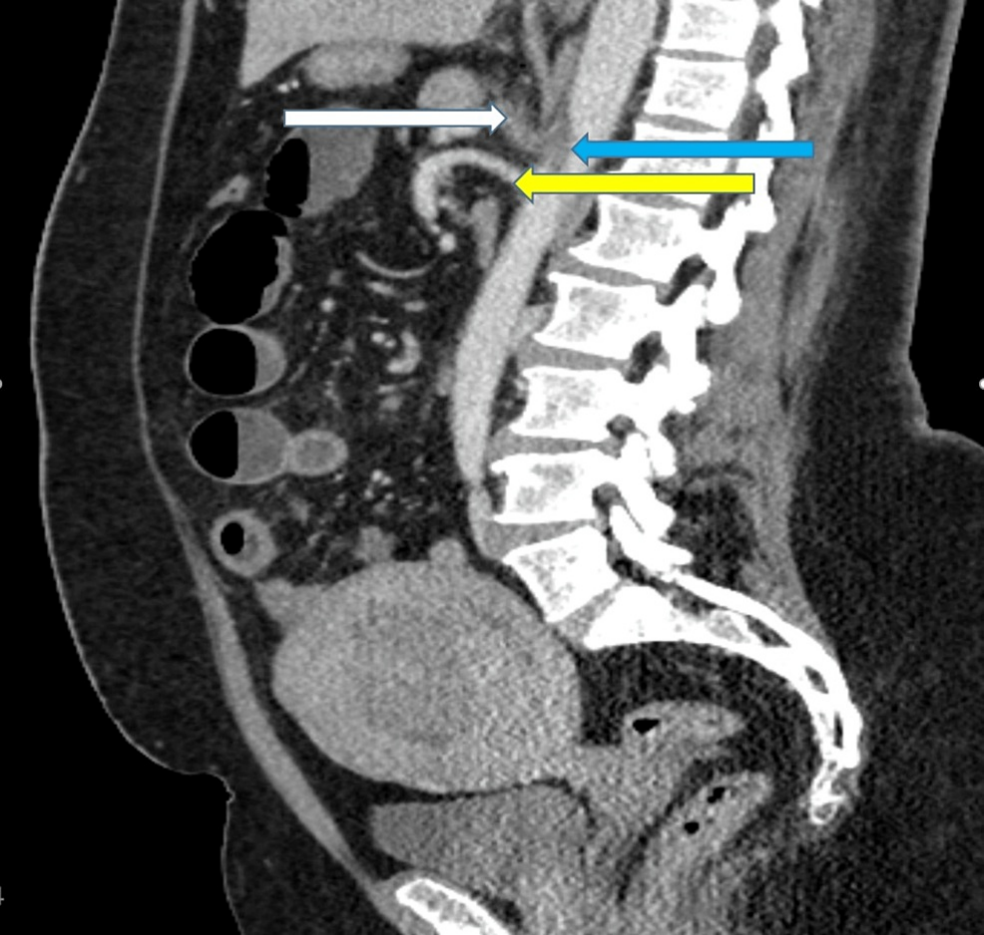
Abdominal CTA image (sagittal view) showing the aortic thrombus (blue arrow) centered on and completely occluding the origin of the celiac trunk artery (white arrow) without involving the superior mesenteric artery (yellow arrow).
